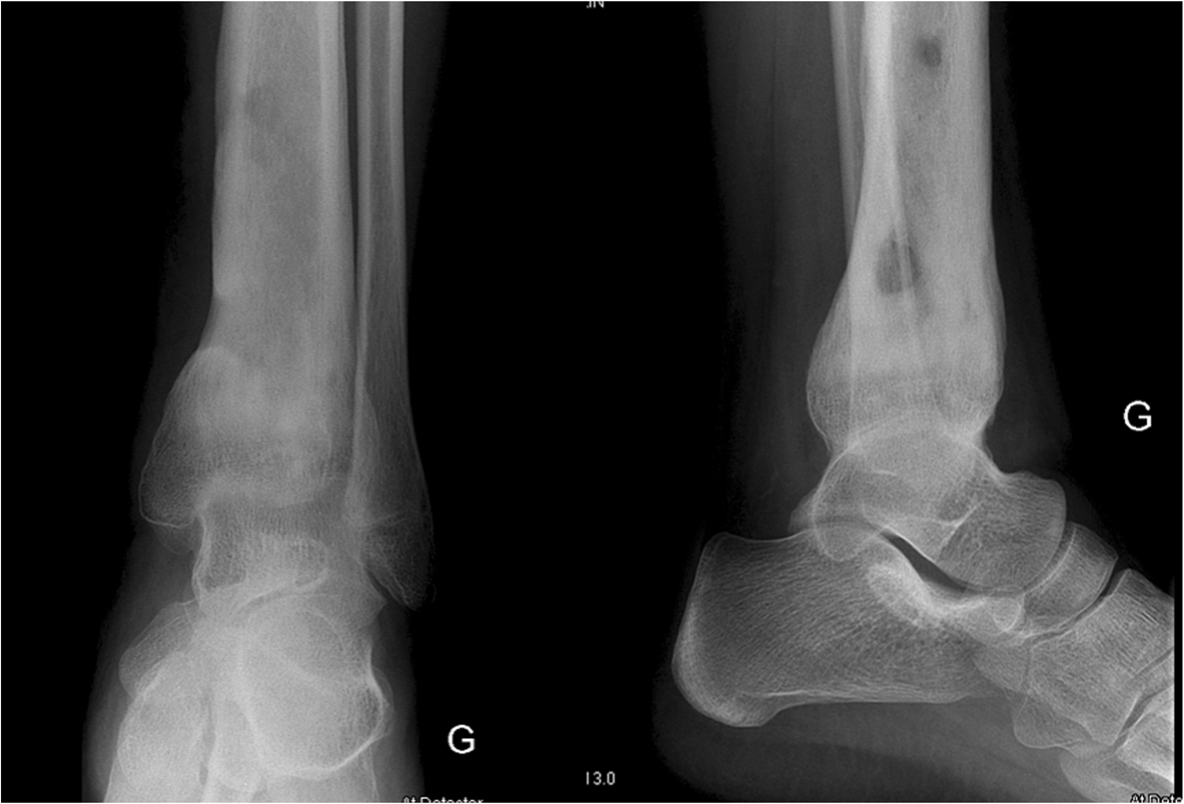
Late control X-rays of the affected tibia three and a half months after disease onset. Extensive bone remodeling consisted of cortical thickening, diffuse sclerosis, anatomical deformation and tibiotalar fusion.
